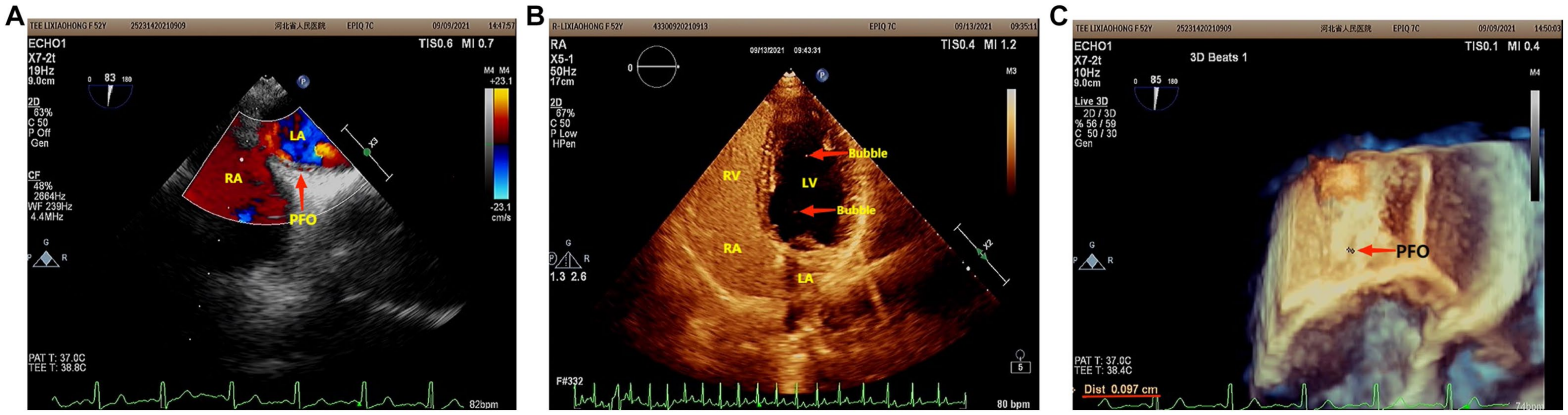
(A) Agitated saline contrast echocardiography showed that the maximum number of bubbles in a single frame was less than 10 bubbles (RLS 1) in three cardiac cycles after the Valsalva maneuver. (B) Two-dimensional echocardiography of the transesophageal heart showed that there was a 0.9 mm wide fissure echo in the middle ovoid fossa of the atrial septum. (C) Three-dimensional echocardiography of the Transesophageal Heart: After the Valsalva maneuver, the width of the ovoid fossa of the atrial septum was approximately 0.9 mm (star-like shunt was occasionally seen).
